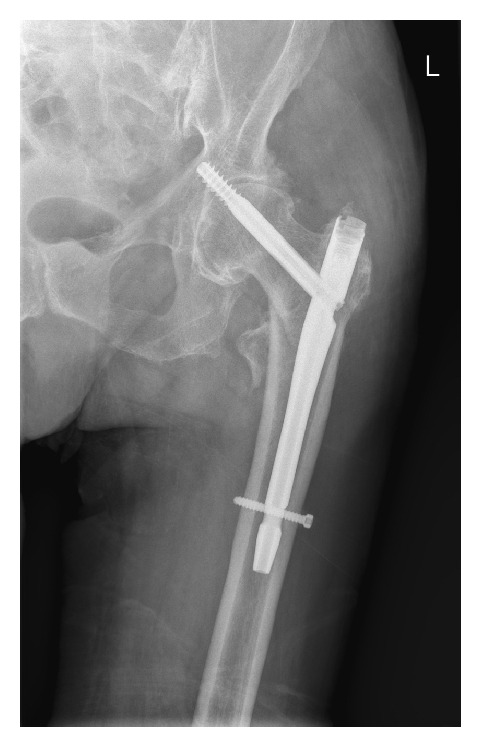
Anterior-posterior X-ray showing medial migration of the lag screw into the acetabulum after 12 weeks.
